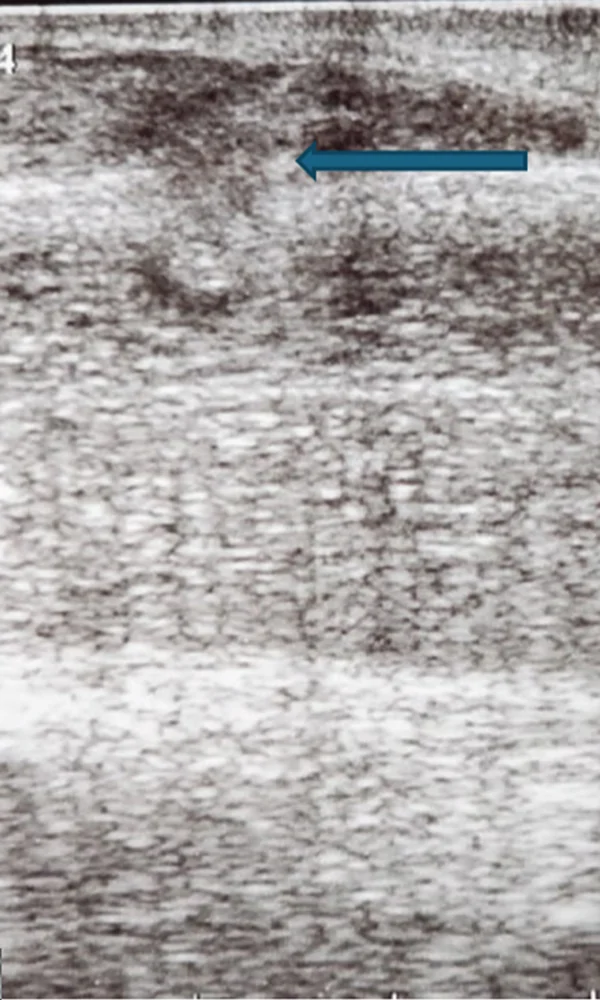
The ultrasound demonstrated a defect in the tunica albuginea of the right corpus cavernosum (the blue arrow).
